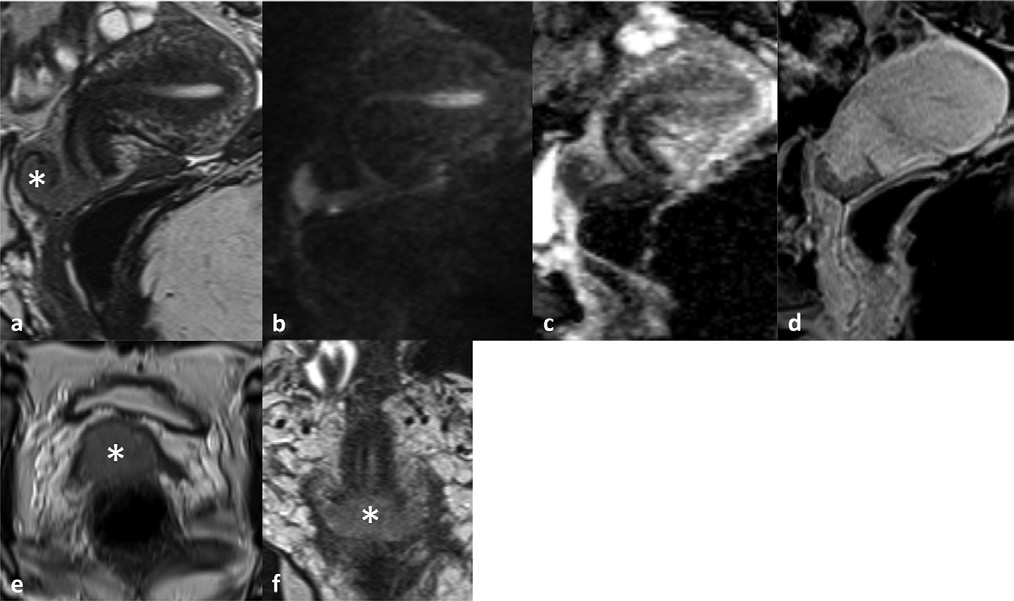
Sagittal (a), axial (e) and coronal oblique (f) T2 images showing a intermediate signal intensity (SI) mass (asterisk) in the anterior lip of the cervix which is high SI on the DWI b-800 images (b) and corresponding low SI on the ADC map (c). The mass shows hypoenhancement relative to the rest of the cervix and myometrium on the delayed phase post-contrast scans (d). ADC, apparent diffusion coefficient; DWI, diffusion-weighted imaging; SI, signal intensity.
